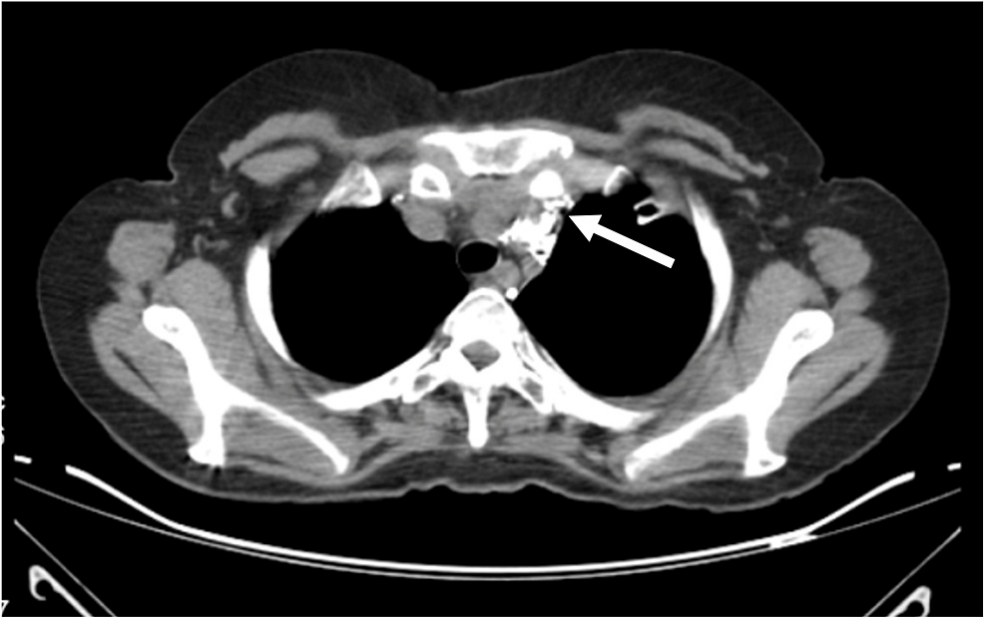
Thoracic duct embolization with Lipiodol®. Lymphatic opacification up to the union of the thoracic duct with the left subclavian vein, with leakage at left thoracic duct level (arrow) (CT chest axial plane).
